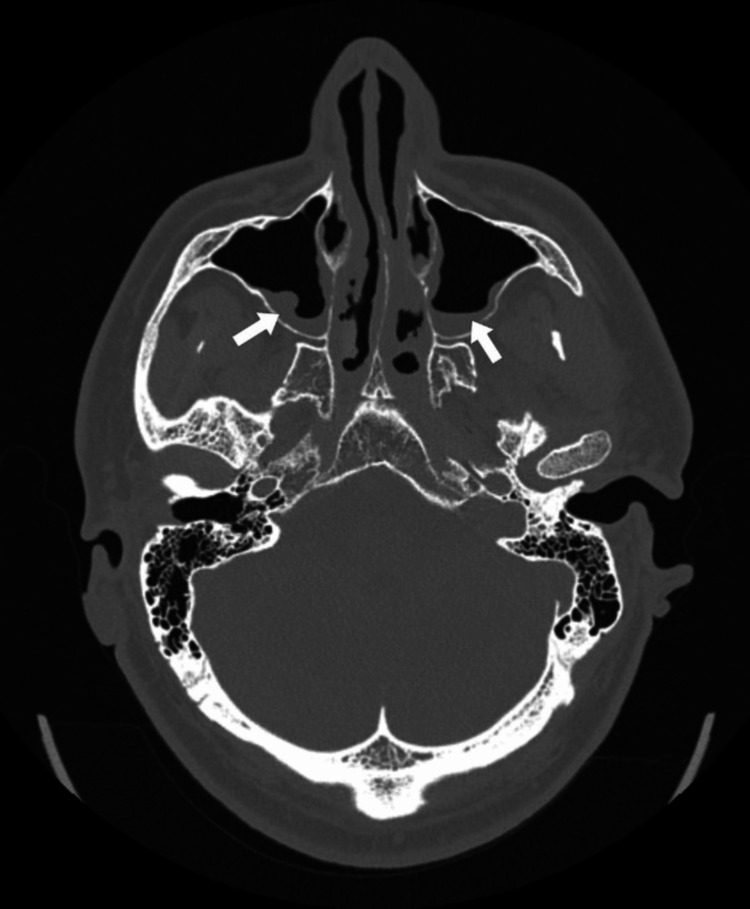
CT scan showing air fluid levels and mucosal thickening CT: computed tomography
